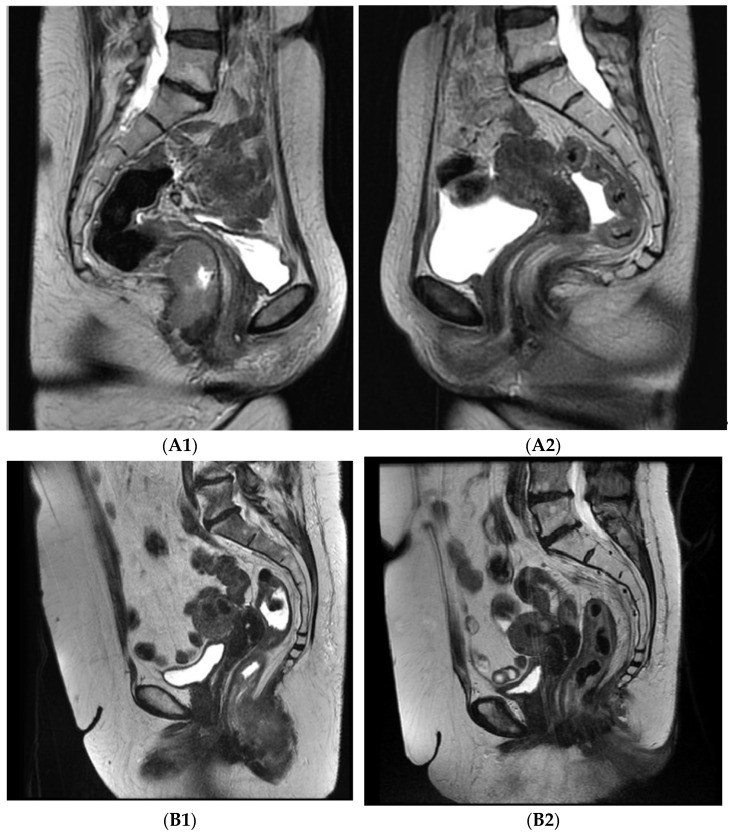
Axial T2 MRI images pre- and post-treatment: A—46 years old female, stage T3N0M0, (A1) pre-treatment, (A2) 8 weeks post-treatment, complete regression; B—62 years old female, stage T3N0M0, (B1) pre-treatment, (B2) 8 weeks post-treatment, partial regression.
